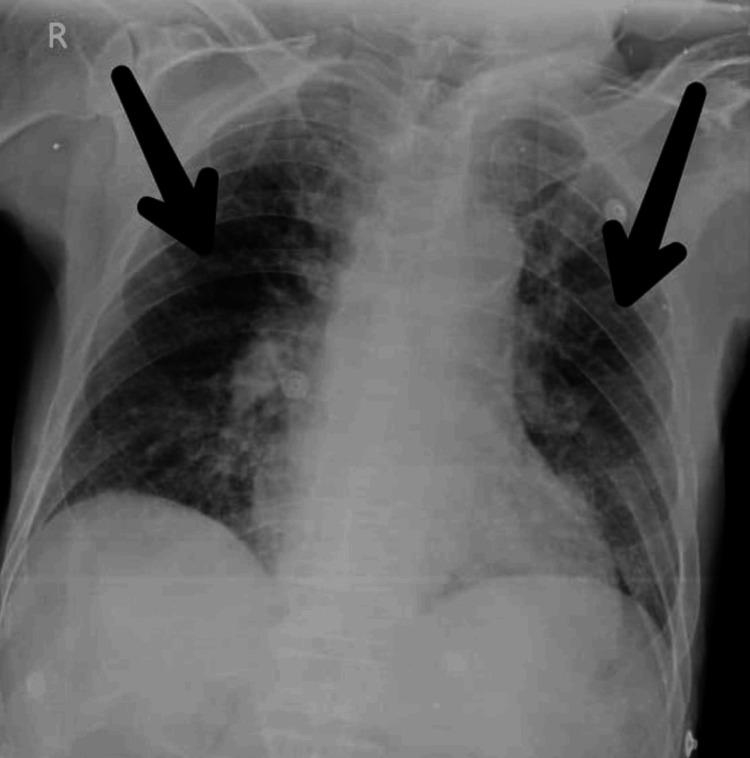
Pre-intervention chest X-ray in PA view PA: posteroanterior The black arrow points at prominent bronchovesicular markings
